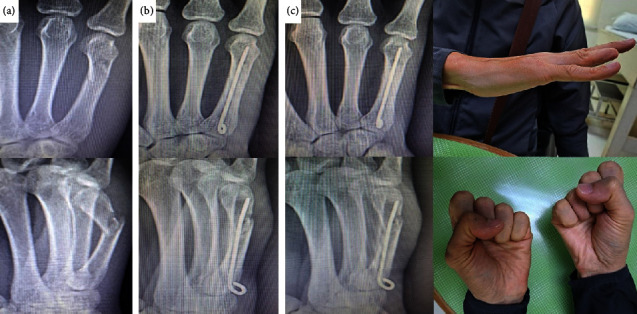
A 51-year-old male with fracture of the fifth metacarpal shaft of the right hand: (a) preoperative radiographs, (b) postoperative radiographs at 2 weeks after surgery, and (c) at 6 weeks postoperative, the patient had dorsal callus bump over the fracture site. He had 15 degrees extension lag, but with full finger flexion. His grip strength was 82.85% and his DASH score was 3.33. He was satisfied with the treatment result and denied further appointment.
